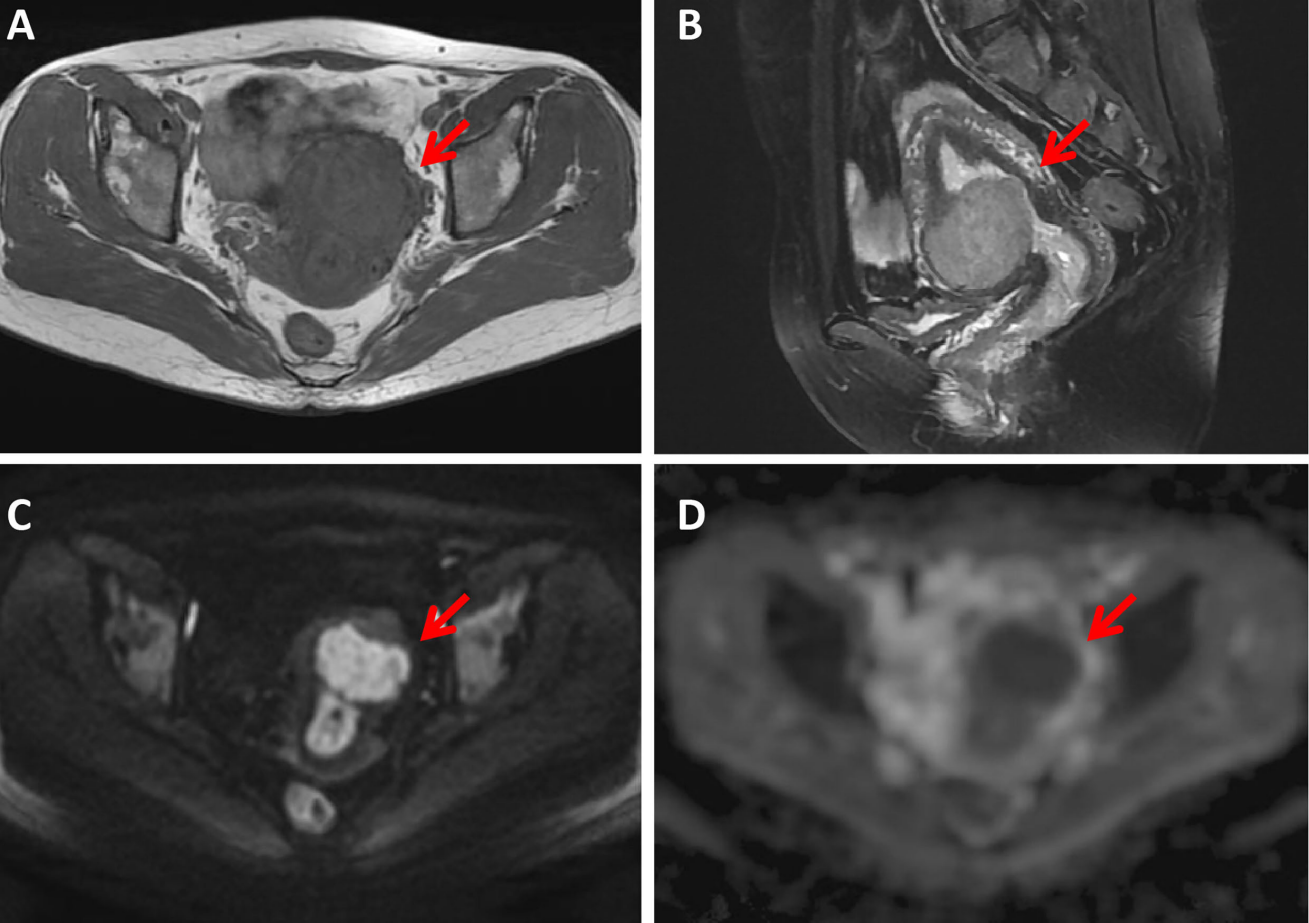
A 43-year-old woman with a cellular leiomyoma. A mass was located in the uterine anterior wall (arrow) with a clear margin, showing isointensity on T1WI (A) and hyperintensity on T2WI images (B). This mass showed high signal intensity on diffusion-weighted MR image (C) with a low ADC value (ADC = 985 ×10^-6^ mm^2^/sec) (D).
